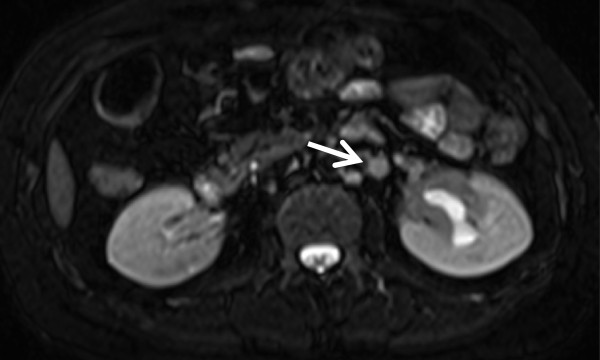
Abdominal T2-weighted magnetic resonance imaging study. This scan shows a low-density renal pelvic mass and hydronephrosis of the left kidney. An enlarged retroperitoneal lymph node (arrow) can be seen.
